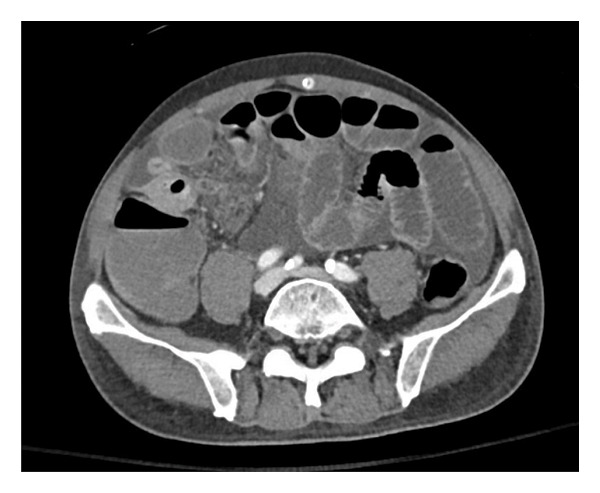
CT of abdomen and pelvis without contrast.
